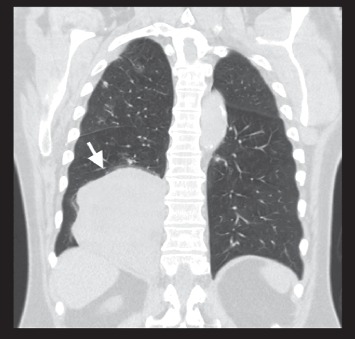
Noncontrast CT chest, coronal view. Visible is a 13.0 × 12.8 × 7.5 cm tumor mass in the right lower lobe of the lung.
